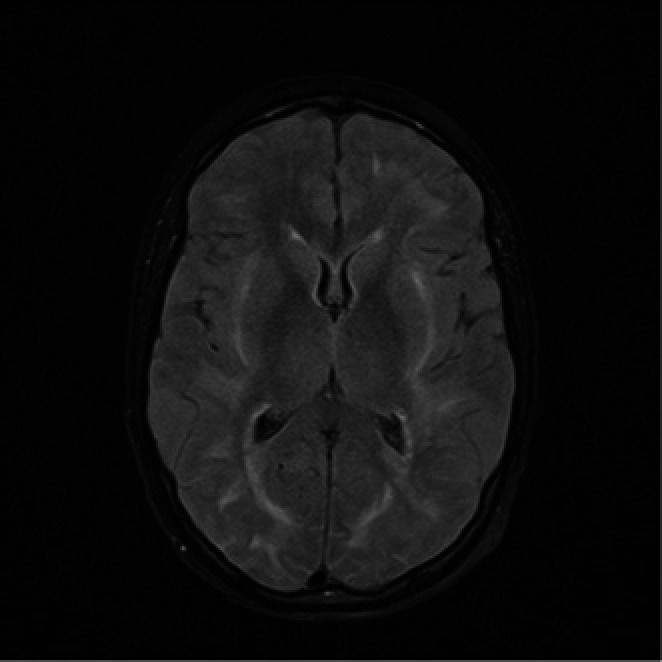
FIGURE 1 Leukoencephalopathy changes on magnetic resonance imaging: T2‐weighted fluid attenuation inversion recovery axial image of the brain demonstrate symmetrical T2 hyperintensity of the deep white matter, most pronounced in the centrum semiovale.
